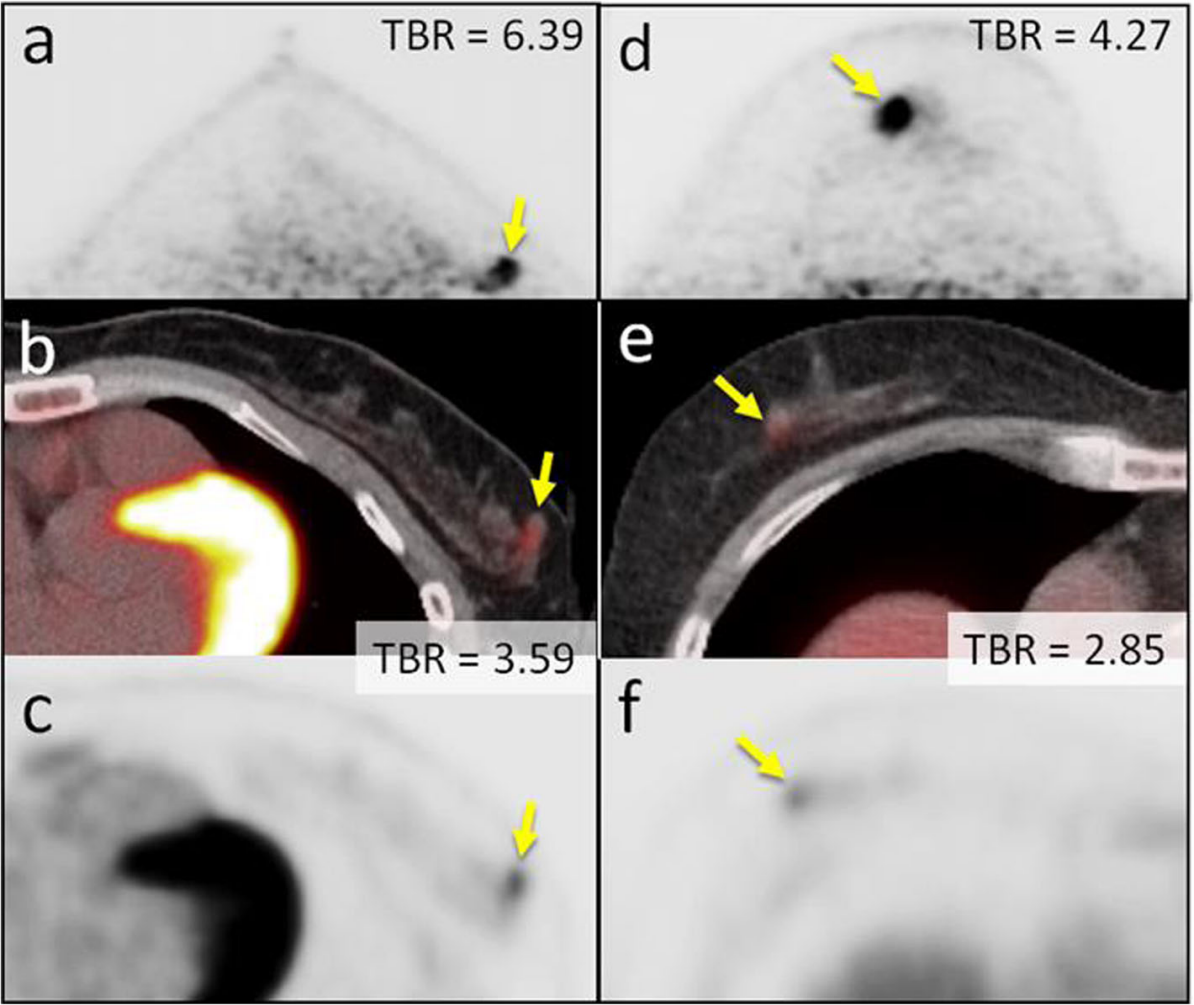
Peripheral and non-peripheral breast cancer images of dbPET scanned in the prone position and whole-body PET/CT scanned in the supine position. Representative clinical images of peripheral (39-year-old woman; clinical size, 12 mm; a–c) and non-peripheral (63-year-old woman; clinical size, 9 mm; d–f) breast cancers on dbPET (a, d), PET/CT (b, e), and PET (c, f) with focal FDG uptake in the background mammary gland tissue. Although the focal uptakes were visualised on both the dbPET and whole-body PET/CT images, it was obvious on dbPET with a higher TBR than that on whole-body PET/CT, regardless of the peripheral or non-peripheral location
